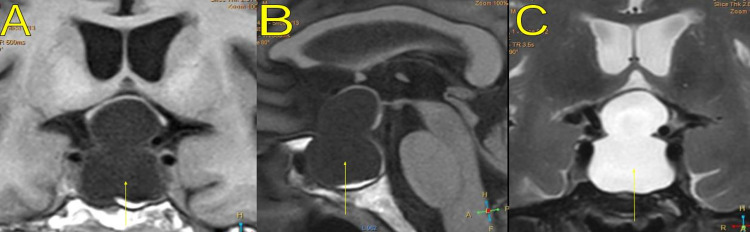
Brain magnetic resonance imaging without contrast performed 16 months later, showing a large sellar/suprasellar arachnoid cyst (yellow arrows), which was stable in size compared to all prior studies: (A) coronal T1-weighted image, (B) sagittal T1-weighted image, (C) coronal T2-weighted image.
